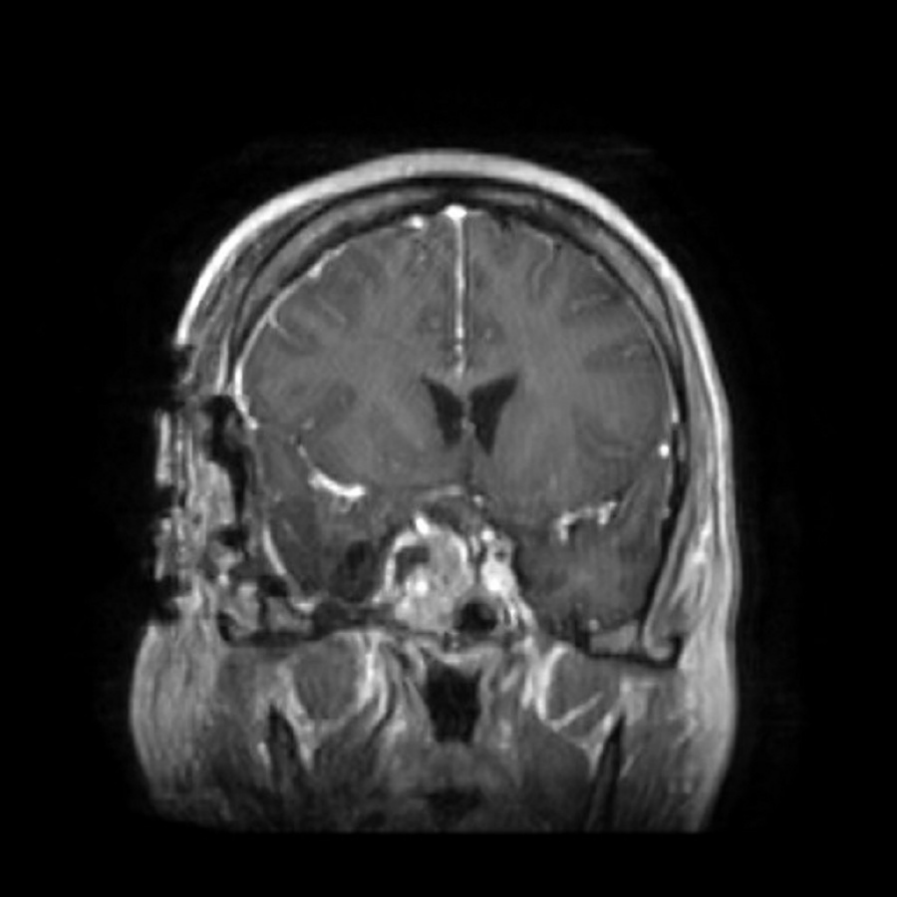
Coronal Post-Contrast MRI Sequences After craniotomy at initiation of TMZ treatment, showing decompression of optic chiasm and debulking of tumor
